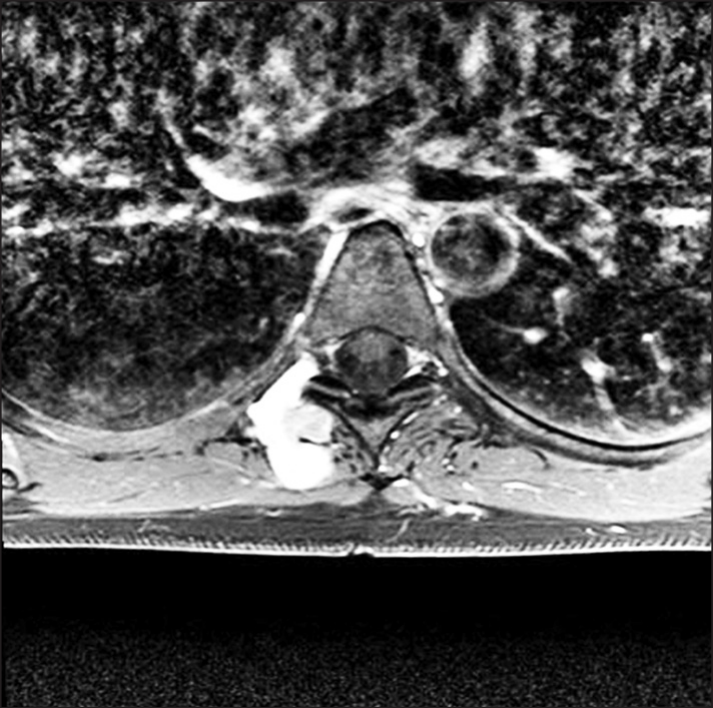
Preoperative T1-axial MRI with contrast shows an enhancing paraspinal mass with foraminal extension
